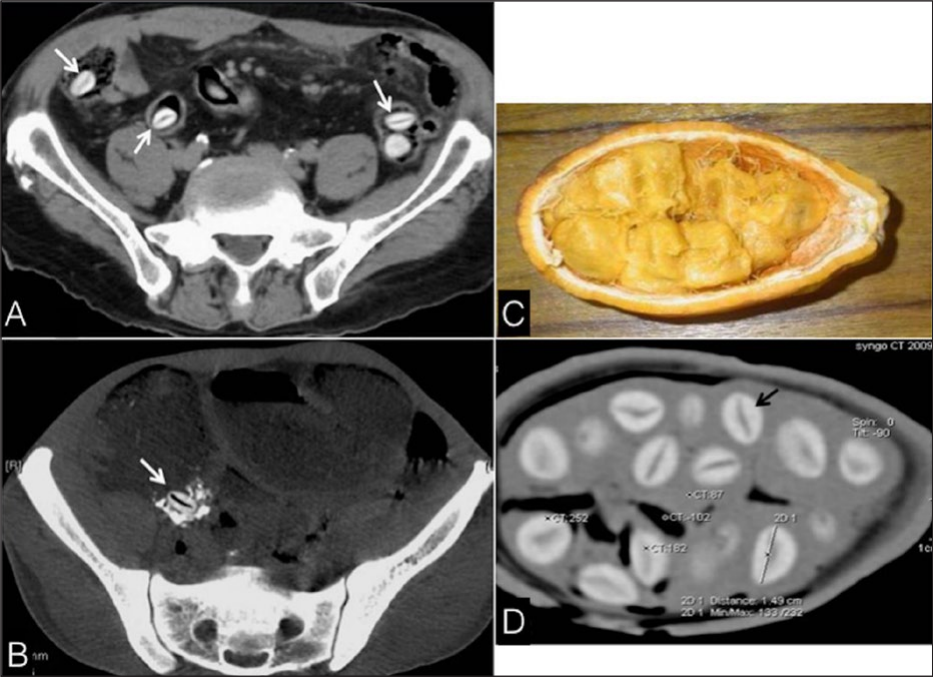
Axial CT images showing (A) SS seeds, incidentally found in the bowel (arrows), and (B) A seed impacted in the ileocecal valve (arrow), responsible for occlusion; Photograph (C) and CT (D) of SS; seed (dark arrow): maximum long diameter: 16 mm, high density of the shell (256 HU) and the midline linear hypodensity (92 HU).
